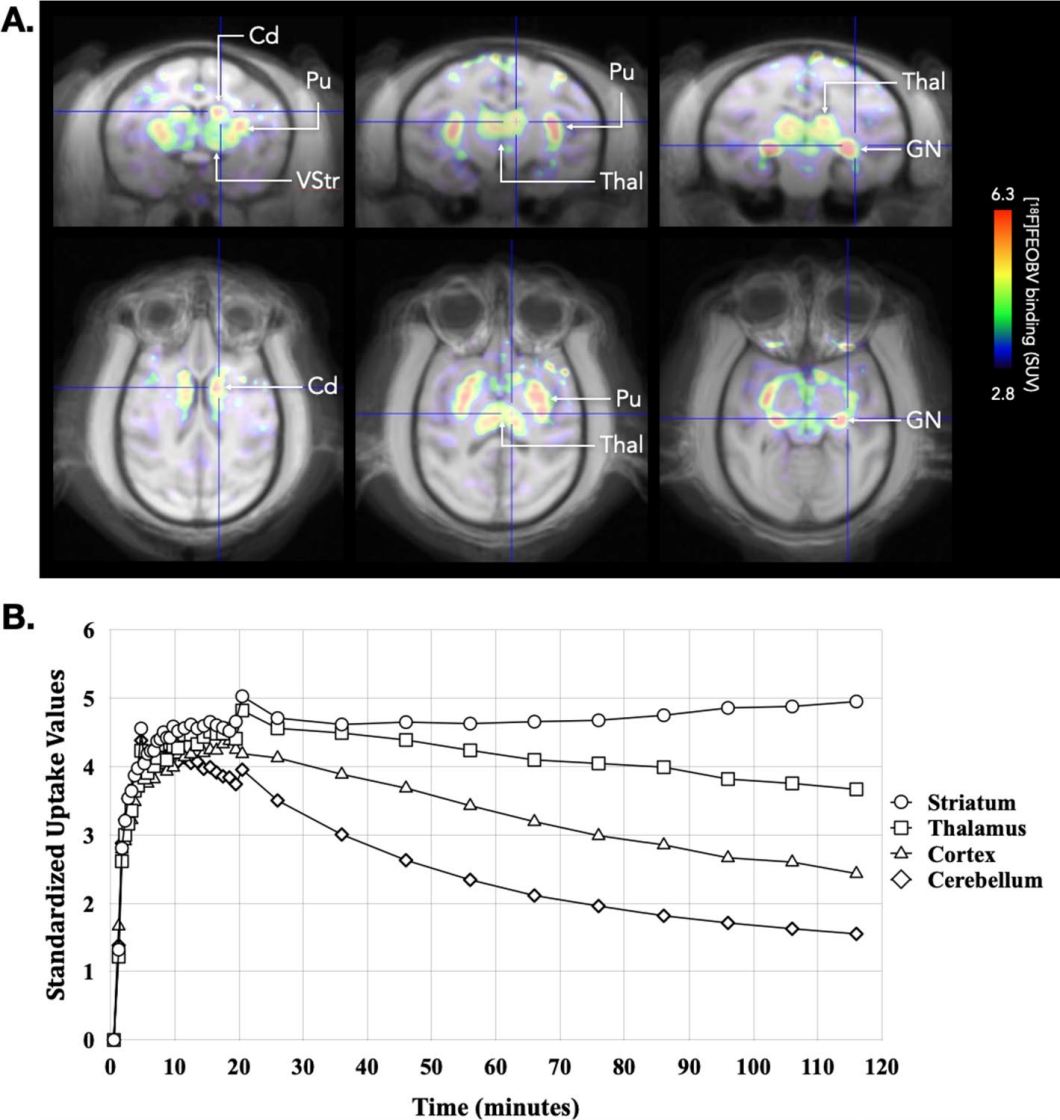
A Fusion between MRI and (-)-[^18^F]FEOBV SUV images (average between 80 and 120 min) showing the (-)-[^18^F]FEOBV binding in the striatum, thalamus and geniculate nuclei on representative coronal and horizontal brain slides. B Time-activity curves of (-)-[^18^F]FEOBV binding in the striatum, thalamus, cortex and cerebellum. *Cd* caudate, *GN* geniculate nuclei, *Pu* putamen, *Thal* thalamus, *VStr* ventral striatum
